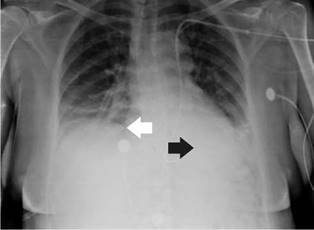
Radiographic control after new placement of transvenous pacemaker through the left subclavian access. The black arrow shows the end of the vascular access located in the right ventricle. The white arrow shows the right atrium.
